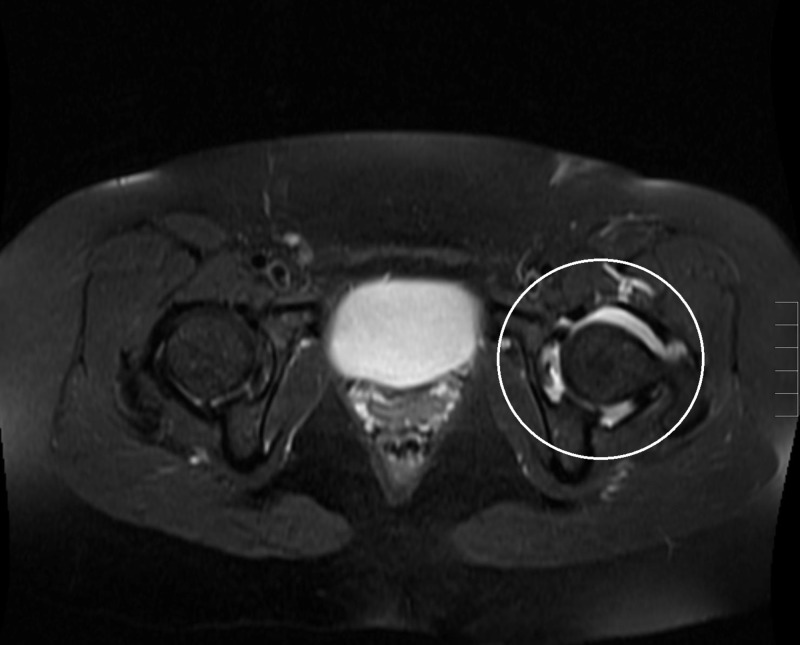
Axial T2 weighted fat-saturated magnetic resonance image of the pelvis A repeated T2 weighted axial fat-saturated magnetic resonance image of the patient's left hip (white circle) revealed the expected appearance, status post intraarticular injection for magnetic resonance arthrography.
